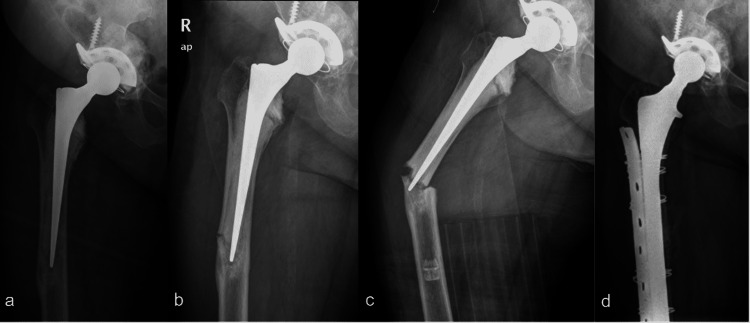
The radiographs from Case 3 a) post-operative total hip arthroplasty before APFF occurred, b) incomplete transverse fracture at lateral cortex with clinical thigh pain at presentation, c) complete fracture progression, d) immediate post-operative revision with long femoral stem augmented with plate and screws with wiring.
